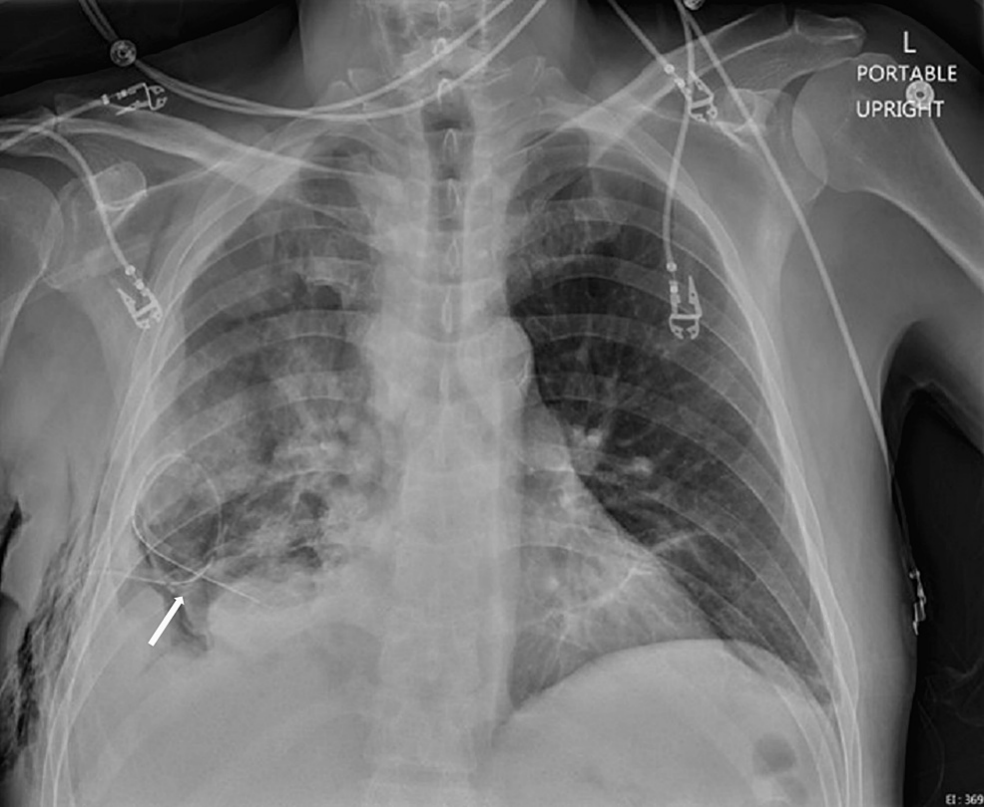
Chest x-ray taken post pleural catheter placement for pleural effusion presentation
